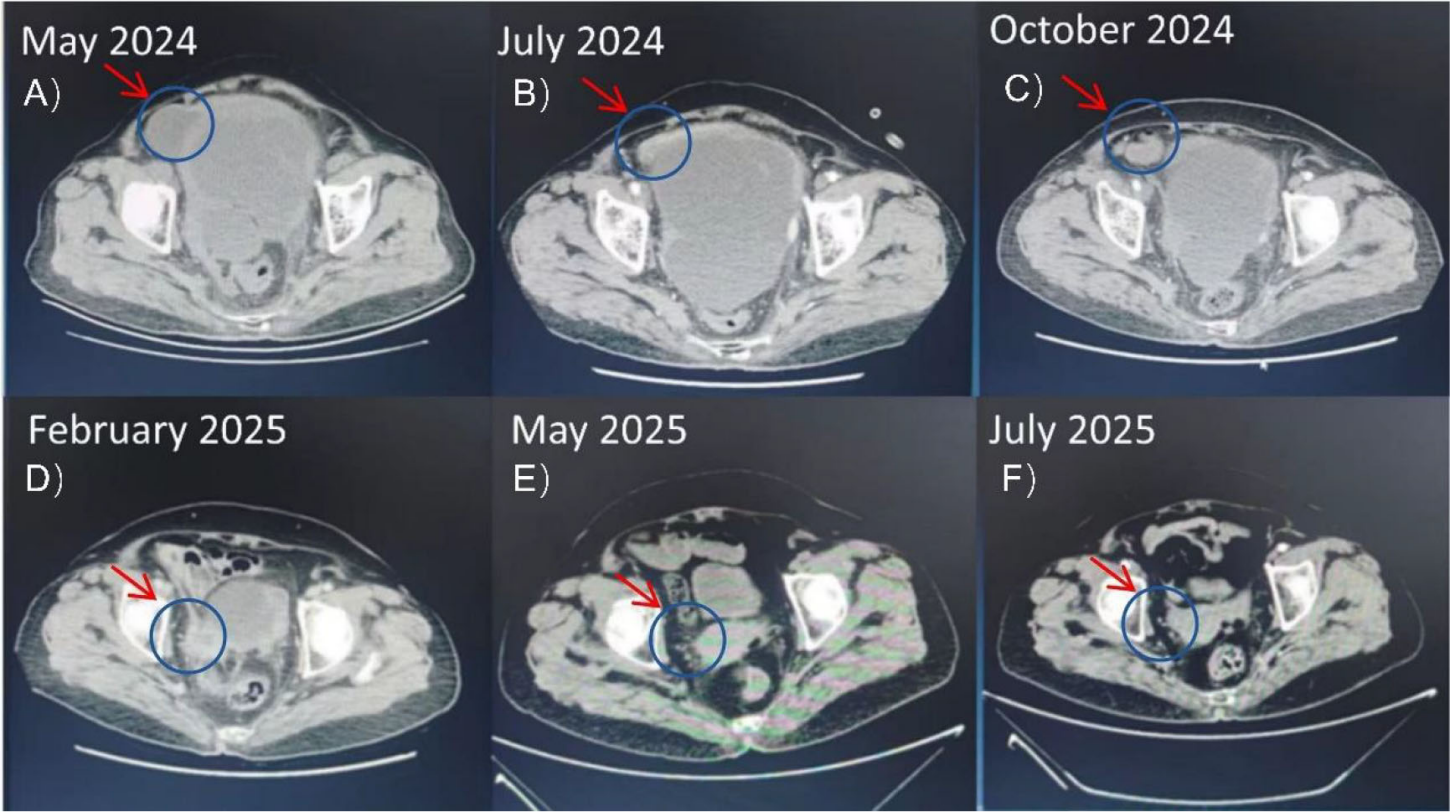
Serial axial CT scans of the pelvis in a patient with peritoneal mesothelioma. The arrows indicate the dominant pelvic mass. (A) Pre-treatment (May 2024) shows a large mass measuring 150 x 160 mm. (B-F) Subsequent images demonstrate a continuous reduction in tumor size following immuno-chemotherapy and maintenance immunotherapy, culminating in the resolution of the measurable lesion by July 2025. .
